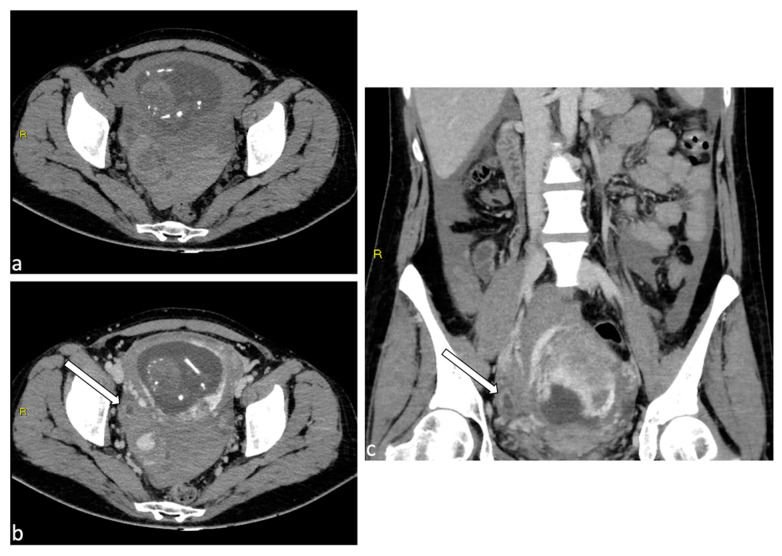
Heterotopic pregnancy. A 35-year-old patient pregnant at 17 weeks with sudden abdominal pain, vaginal bleeding, and hypotension. Transabdominal ultrasound documented viable intrauterine fetus and hemoperitoneum. Given the unavailability of MRI, an urgent CT scan was performed, which showed abundant hemoperitoneum (a), intact gravid uterus and a cystic mass with peripheral enhancement within the right fallopian tube (b,c, arrows). An emergency laparoscopy was performed and revealed a congested and swollen right salpinx, site of an ectopic pregnancy. A right salpingectomy was performed. The patient was then discharged and successfully completed the intrauterine pregnancy.
